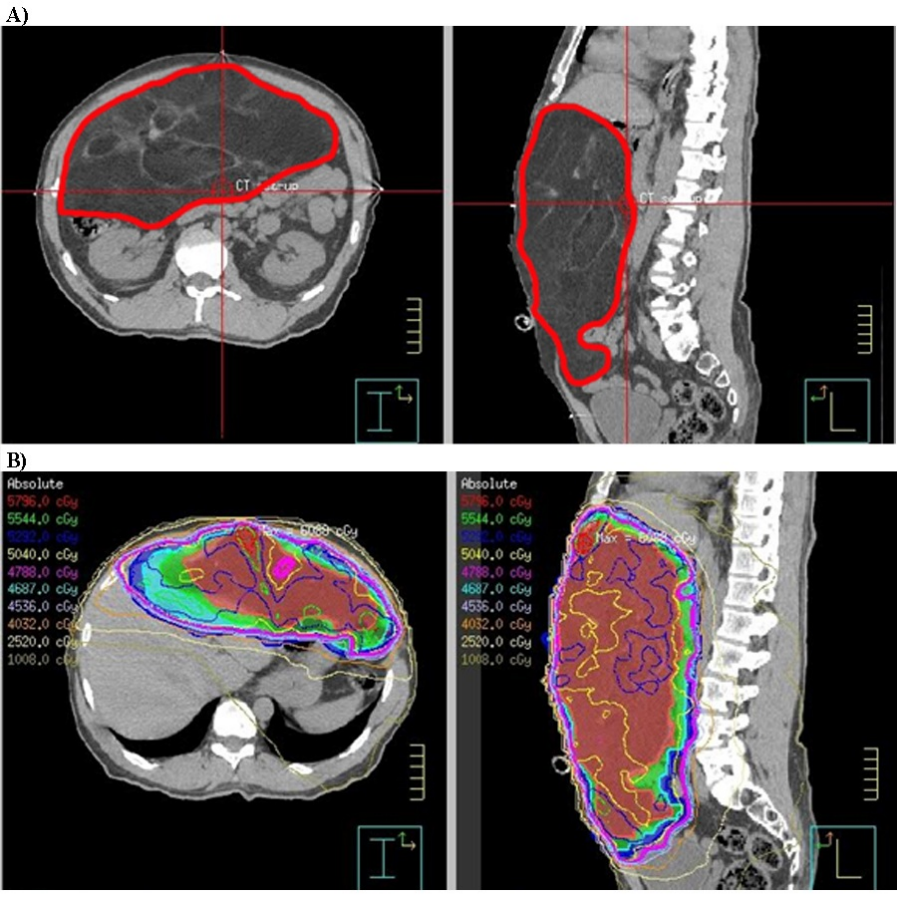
CT scan and radiotherapy plan of a retroperitoneal sarcoma patient Sample computed tomography (CT) scan (A) of a patient with a primary retroperitoneal sarcoma (contoured in red) and the intensity modulated radiotherapy (IMRT) plan and isodose curves (B).
